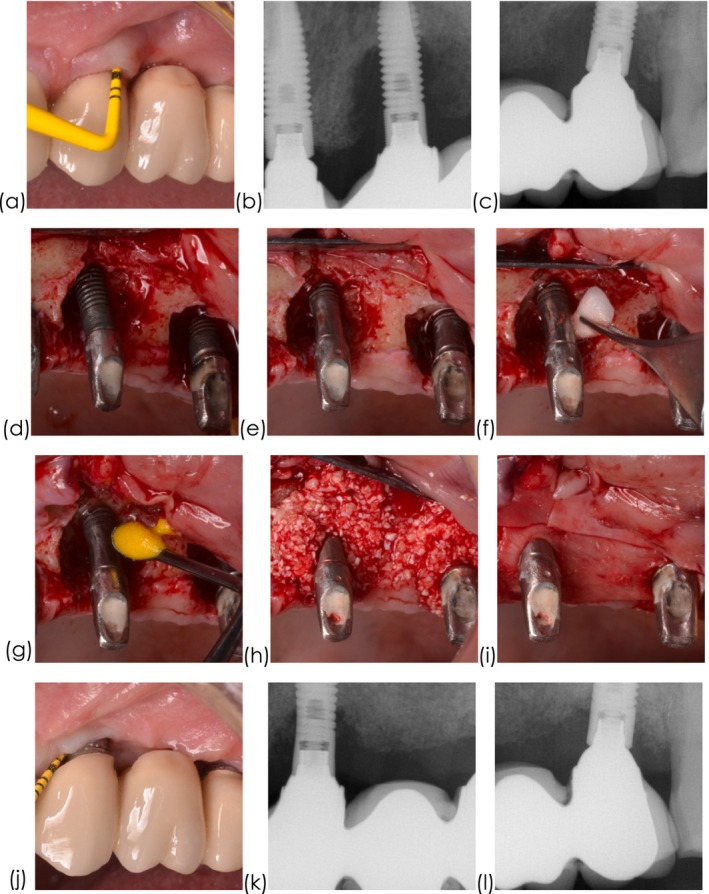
Representative case of FLIP. (a) clinical presentation following non‐surgical therapy, (b–c) periapical radiographic displaying advanced bone loss, (d) intra‐operative presentation showing combined defects, (e) implantoplasty along the supracrestal and intrabony components, (f) chemical decontamination applying hydrogen peroxide, (g) pharmacological decontamination using tetracycline chlorhydrate, (h) mixture of autogenous bone with xenograft, (i) collagen membrane is used to enhance graft stability, (j) clinical outcome showing disease resolution, (k–l) radiographic marginal bone level gain.
